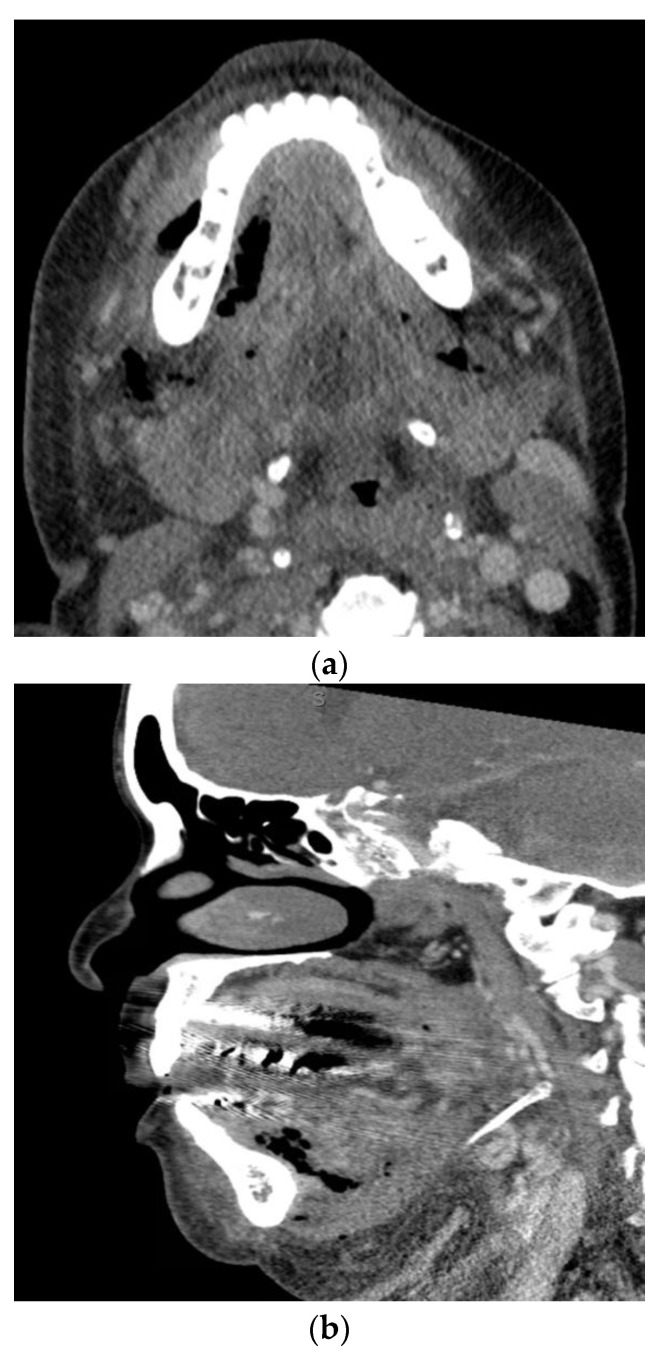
Axial (a) and sagittal (b) computed tomography scan views of a patient with Ludwig’s angina.
